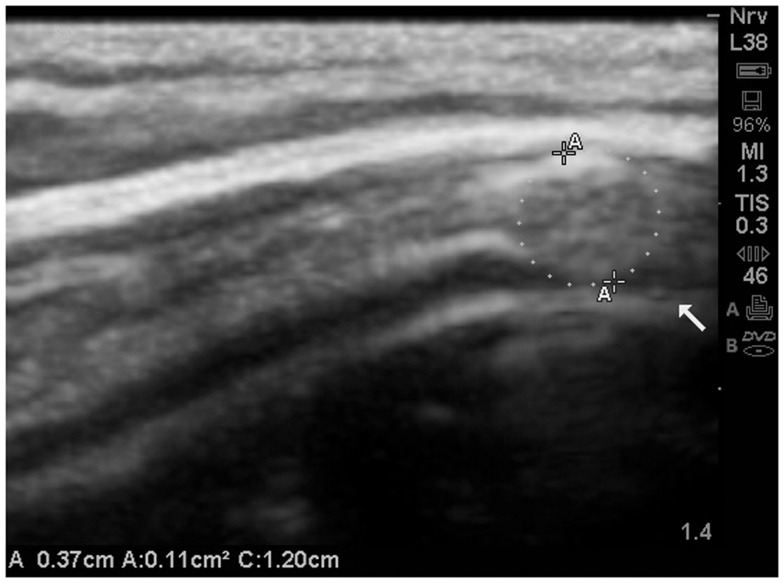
The subarachnoid space at the gyral summit demonstrates abrupt closure secondary to a mass effect within the meninges. Further, the gyral summit can be observed to flatten somewhat by the effect of meningeal mass. This is a presumed meningioma and most likely benign. The patient has been referred for MRI and further consultation. MRI is not presently available.
